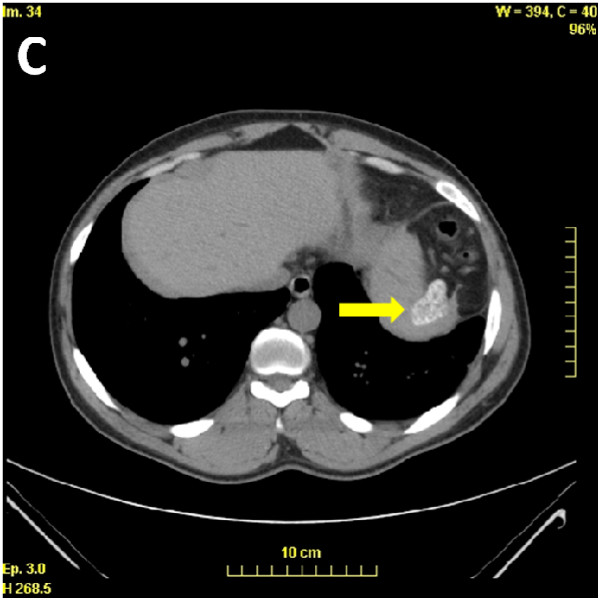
Abdominal CT-scan identify a small calcified spleen (yellow arrow).
